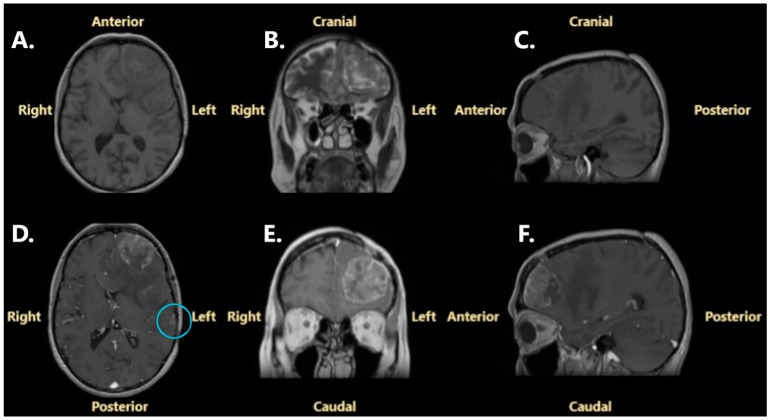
Brain MRI performed in May 2024: (A)—non-contrast T1-weighted Fast Spoiled Gradient-Echo (FSPGR) sequence, axial projection; (B)—non-contrast T2-weighted Fast Spin Echo (FSE) sequence, coronal projection; (C)—non-contrast T1-weighted Multiplanar Reconstruction (MPR), sagittal projection; (D)—contrast enhancement on T1-weighted FSPGR, axial projection; (E)—contrast enhancement on T1-weighted MPR T1, coronal projection; (F)—contrast enhancement on T1-weighted MPR, sagittal projection. The blue circle surrounds the left frontoparietal extra-axial tumor.
